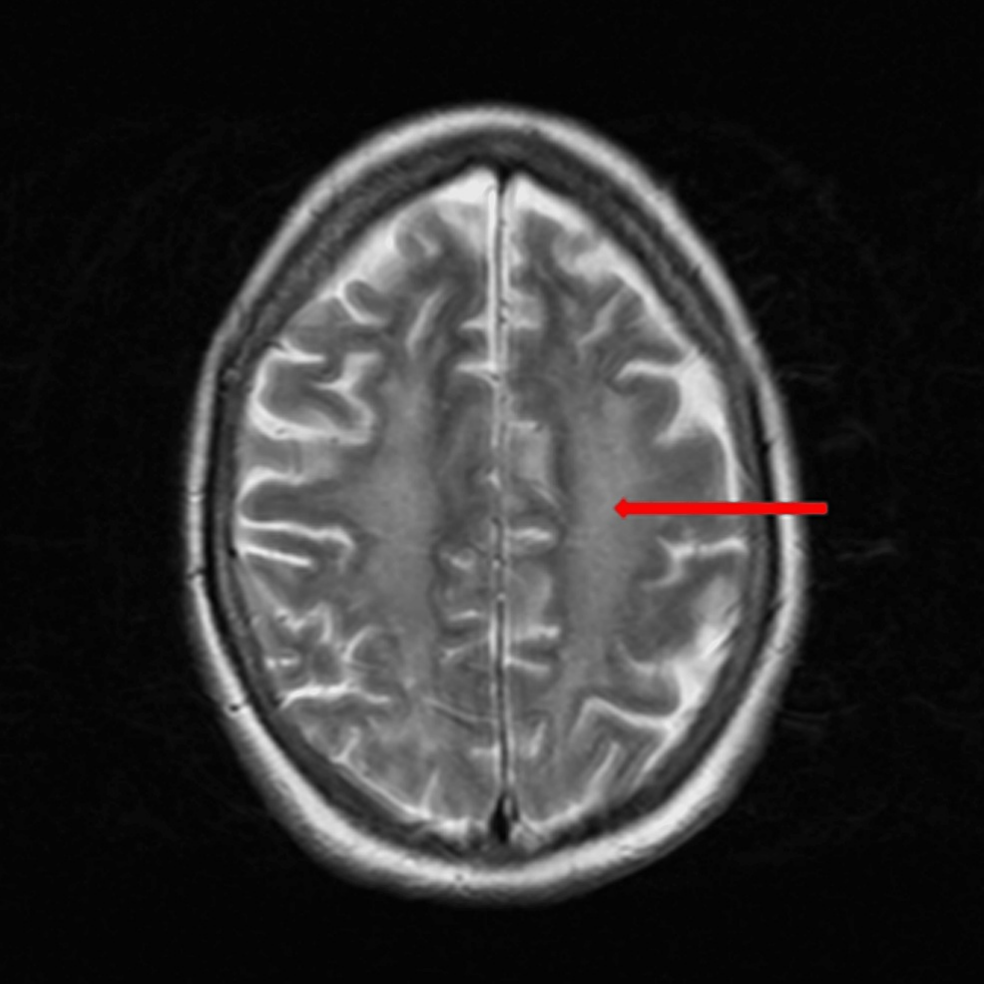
Magnetic resonance imaging (MRI) AX T2 showing diffuse bilateral cerebral white matter changes (red arrow) involving the frontal, parietal, occipital, and temporal lobes.
